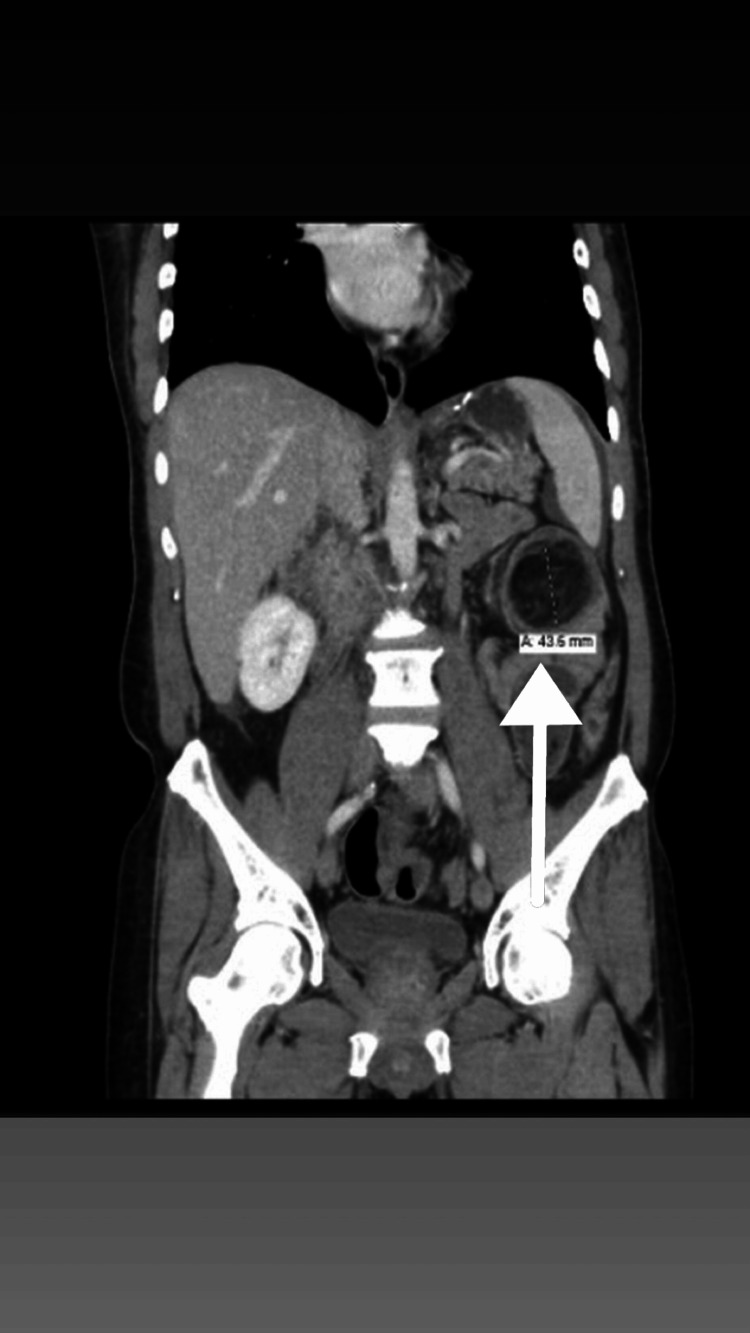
Coronal CT demonstrating cecocolic intussusception Abnormal orientation of the cecum is seen, with concentric alternating layers of bowel and fat directed from the right side of the abdomen toward the left upper quadrant. This image is highly suggestive of cecocolic intussusception (cecum into transverse colon)
